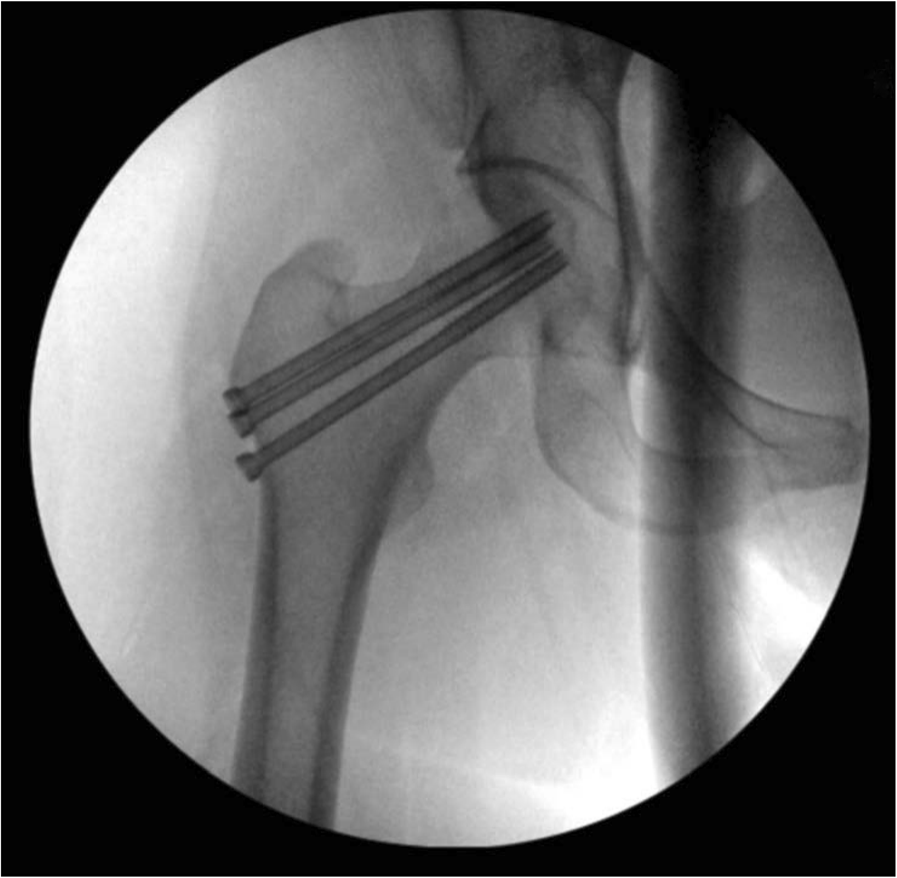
Intraoperative fluoroscopic radiograph of the right hip demonstrating screw fixation of a femoral neck stress fracture
